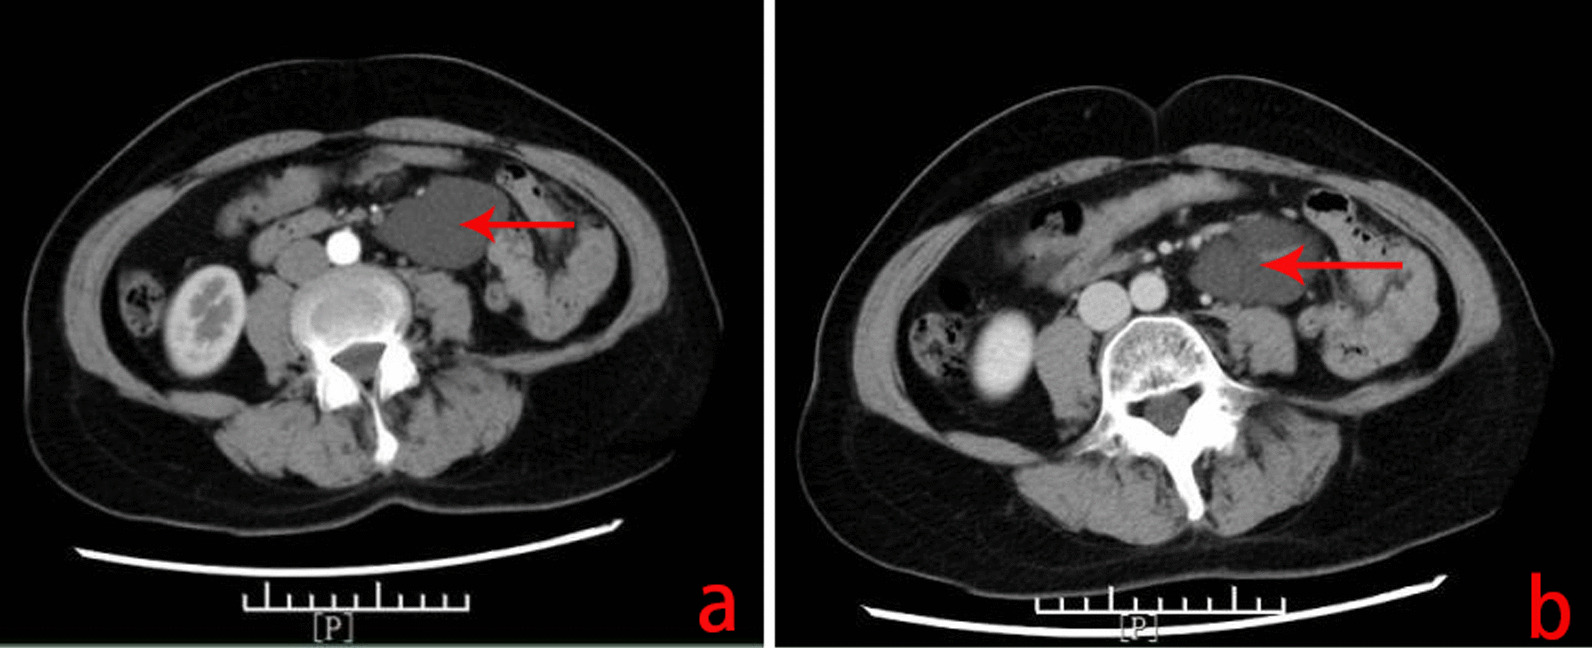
Enhanced CT scan showing an abdominal low-density mass (arrows) of 5.9 cm × 4.1 cm. The CT value was approximately − 2 to − 4 HU, and there was no significant enhancement in the arterial phase (a) but slight enhancement in the venous phase (b) (Case 2)
